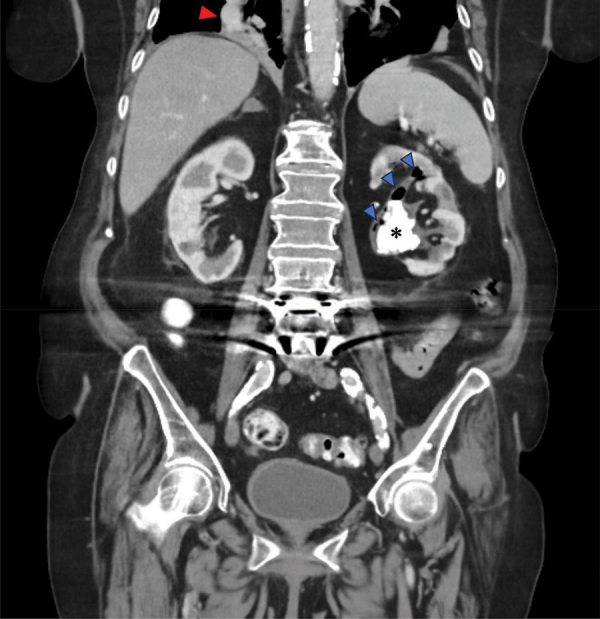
CT abdomen - coronal plane.
